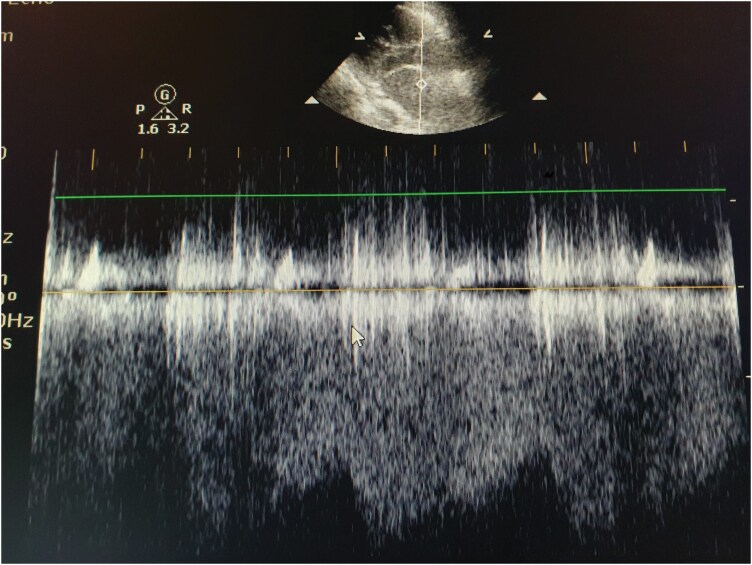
Continuous-wave Doppler recording confirming flow between a high-pressure and a low-pressure chamber.
